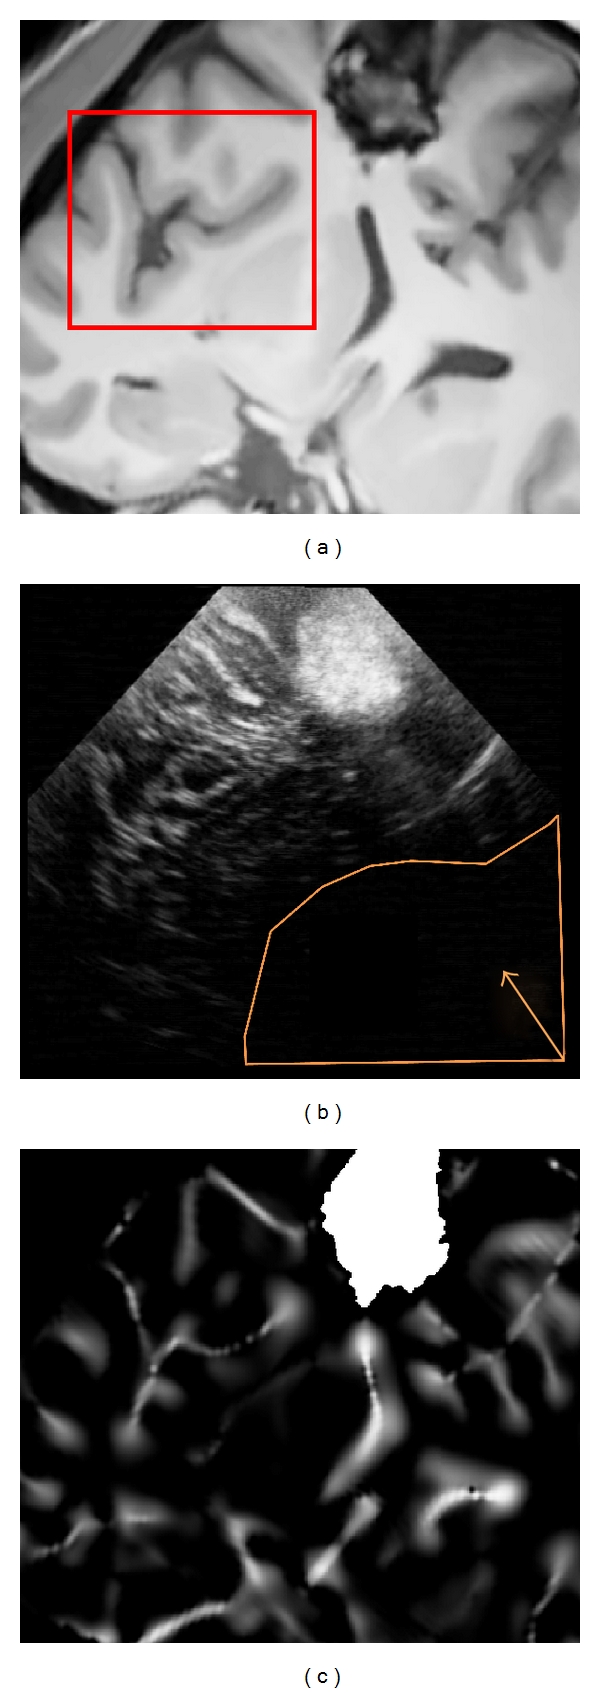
Patient 2. (a) The denoised MR image corresponding to US image (b). (c) The probability map based on *MLvv* operator and extracted from denoising MR image. The preoperative MR image is resliced with the registration matrix provided by the neuronavigation system. The matching is not perfect due to the initial error, estimated around 8.5 mm by the experts for this patient. The red box shows a sulcal area where the matching between US image and the *MLvv*-based probability map is visually high. A large acoustic shadow is visible in US around the ventricle area (indicated in orange on the US image).
